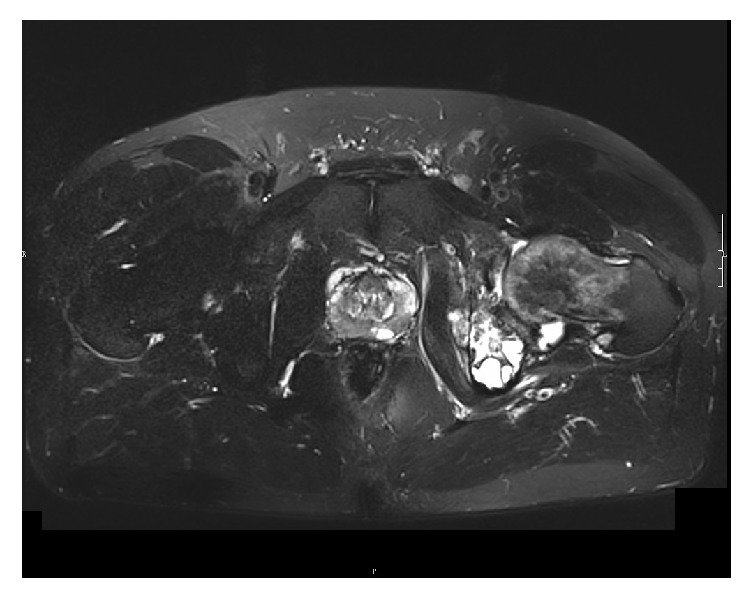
MRI: T2-weighted sequence.
